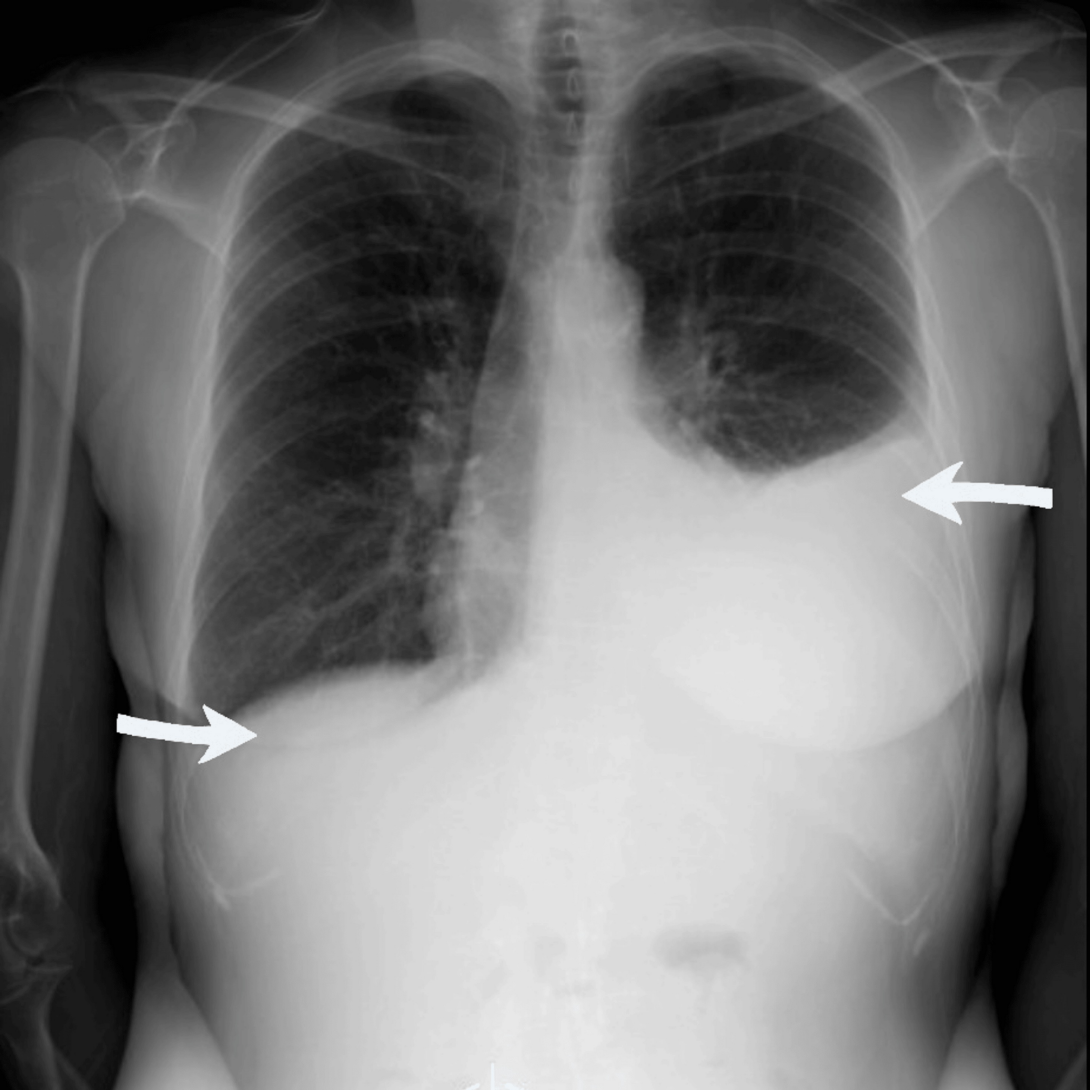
Chest X-ray at time of presentation demonstrating a large left and a smaller right pleural effusion, as denoted by the arrows While this finding did not necessarily raise clinical suspicion for amyloidosis, it did provide a rationale for the presenting shortness of breath and also led to the further workup that eventually led to the confirmatory diagnosis.
